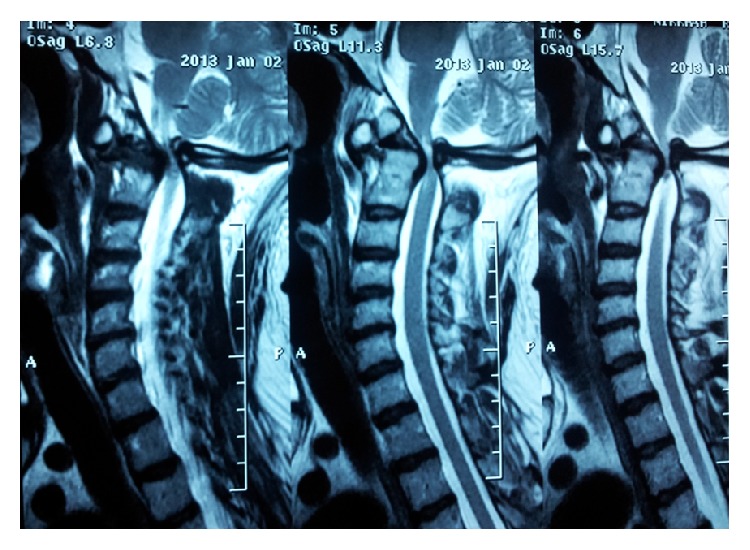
T1-weighted sagittal MR images showing os odontoideum, severe narrowing, and a hyperintense signal at cervicomedullary junction indicating myelopathy.
